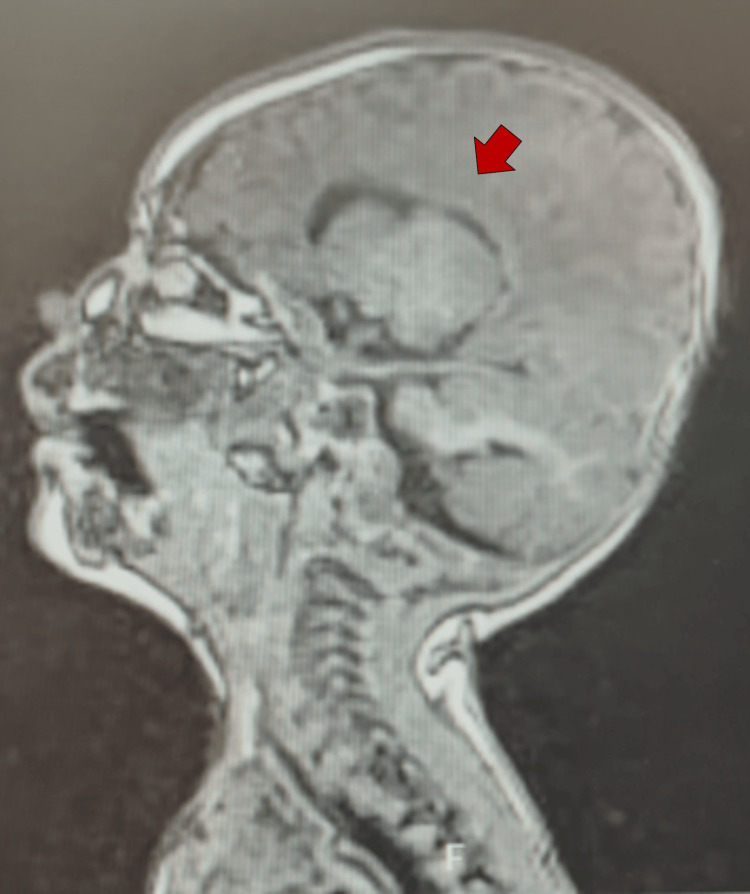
MRI scan of the brain Parasagittal MRI scan demonstrating agenesis of the corpus callosum, characterized by the absence of normal callosal fibers (red arrow). The image also reveals increased space within the posterior fossa, possibly indicative of a Blake pouch cyst.
